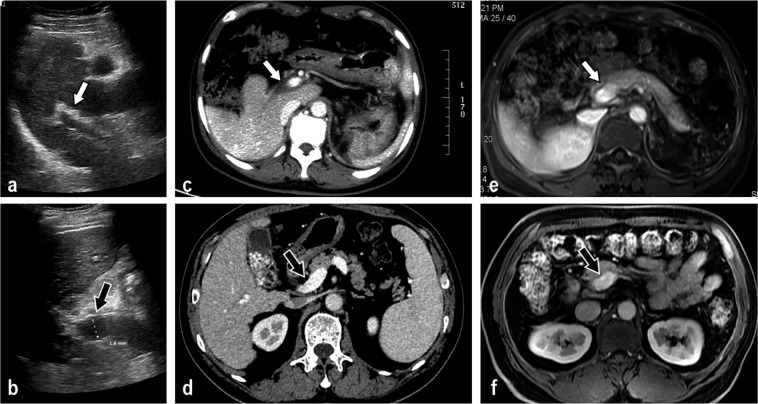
The change in main portal vein wall thickness in idiopathic portal hypertension (IPH) and cirrhosis patients based on ultrasound, CT and MR imaging (TR 4.8, TE 2.4). (a) The ultrasound image shows the thickened and rough vascular wall (white arrow) in an IPH patient. (b) The ultrasound image shows the thin vascular wall (black arrow) and dilated lumen of the main portal vein in a cirrhosis patient. (c) The contrast-enhanced CT image shows the thickened and rough lowly enhanced vascular wall (white arrow) in the delay phase in an IPH patient. (d) The contrast-enhanced CT image shows no significant change of the main portal vein vascular wall in the delay phase in a cirrhosis patient. (e) The contrast-enhanced MR T1WI image shows the thickened vascular wall in the delay phase (white arrow) in an IPH patient. (f) Contrast-enhanced MR image shows no significant change in the main portal vein vascular wall in the delay phase in a cirrhosis patient.
